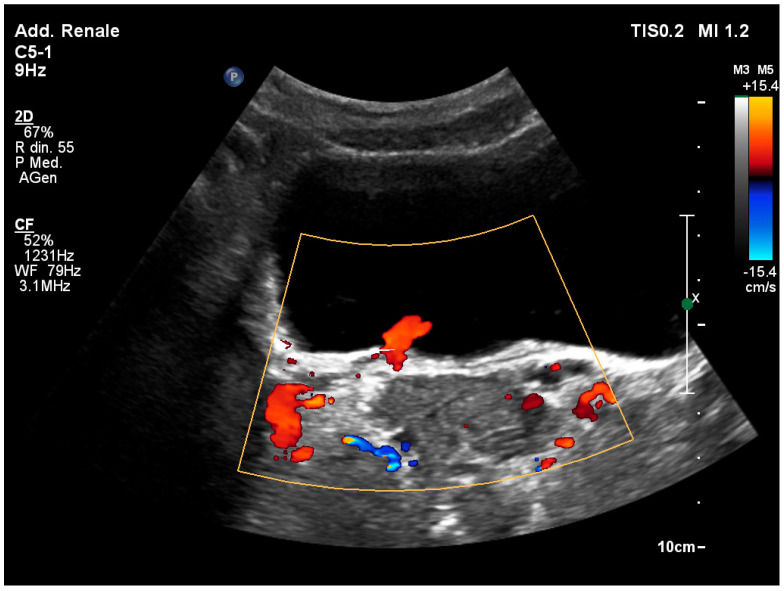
While the right ureteral jet is visible as a color Doppler stream, the left ureteral jet is not viewable.
